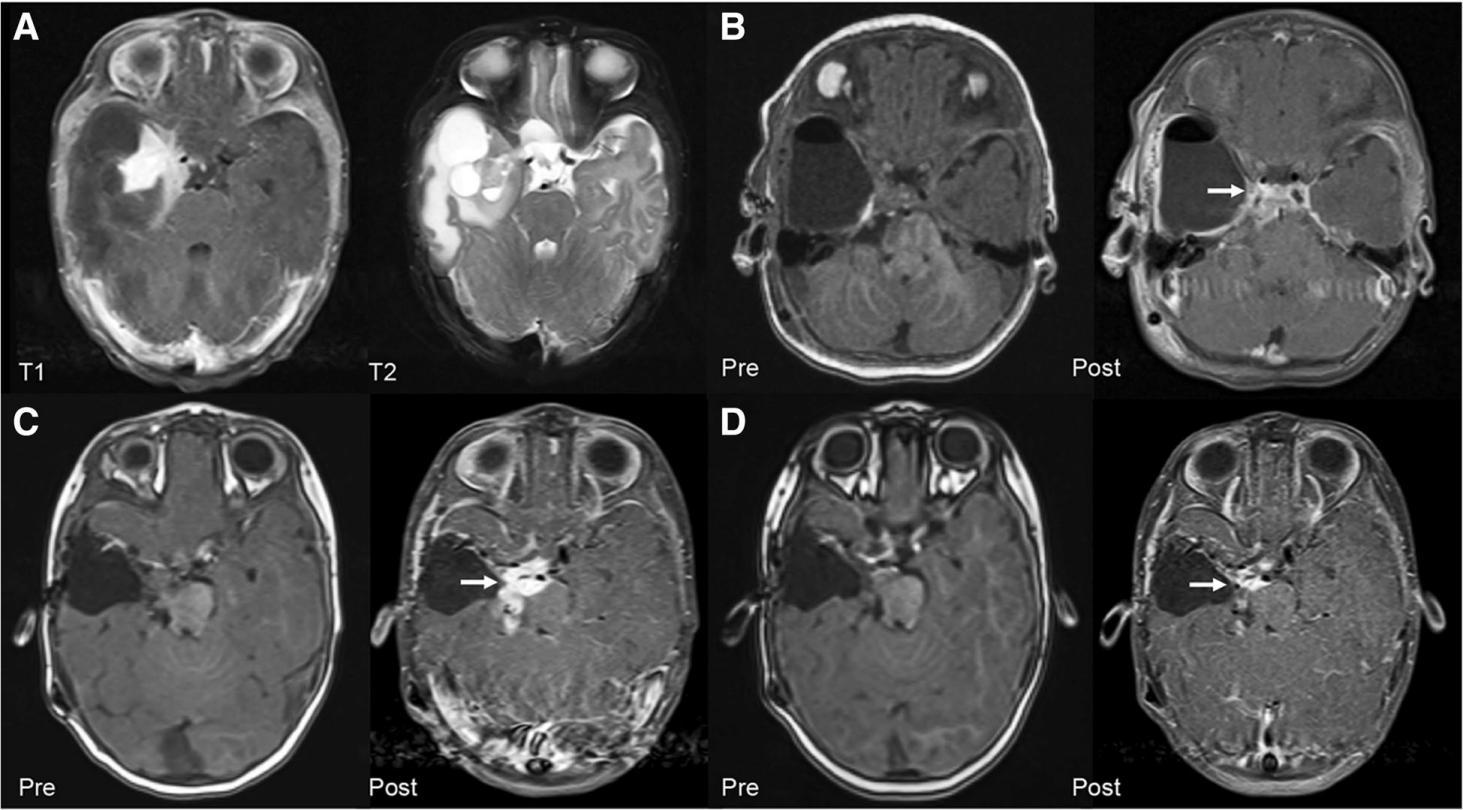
Radiologic findings. a (T1-Weighted Postcontrast and T2-Weighted Axial Magnetic Resonance Imaging), Large right inferomedial temporal solid-multicystic mass with a postcontrast enhancing component. b (T1-Weighted Pre and Postcontrast Axial Magnetic Resonance Imaging), Three-month postoperative leptomeningeal spread involving the upper brainstem. c (T1-Weighted Pre and Postcontrast Axial Magnetic Resonance Imaging), Eight-month postoperative progression of leptomeningeal involvement despite standard chemotherapy. d (T1-Weighted Pre and Postcontrast Axial Magnetic Resonance Imaging), Fourteen-month postoperative decrease in residual tumor after 6 months of BRAF-MEK inhibitor therapy
